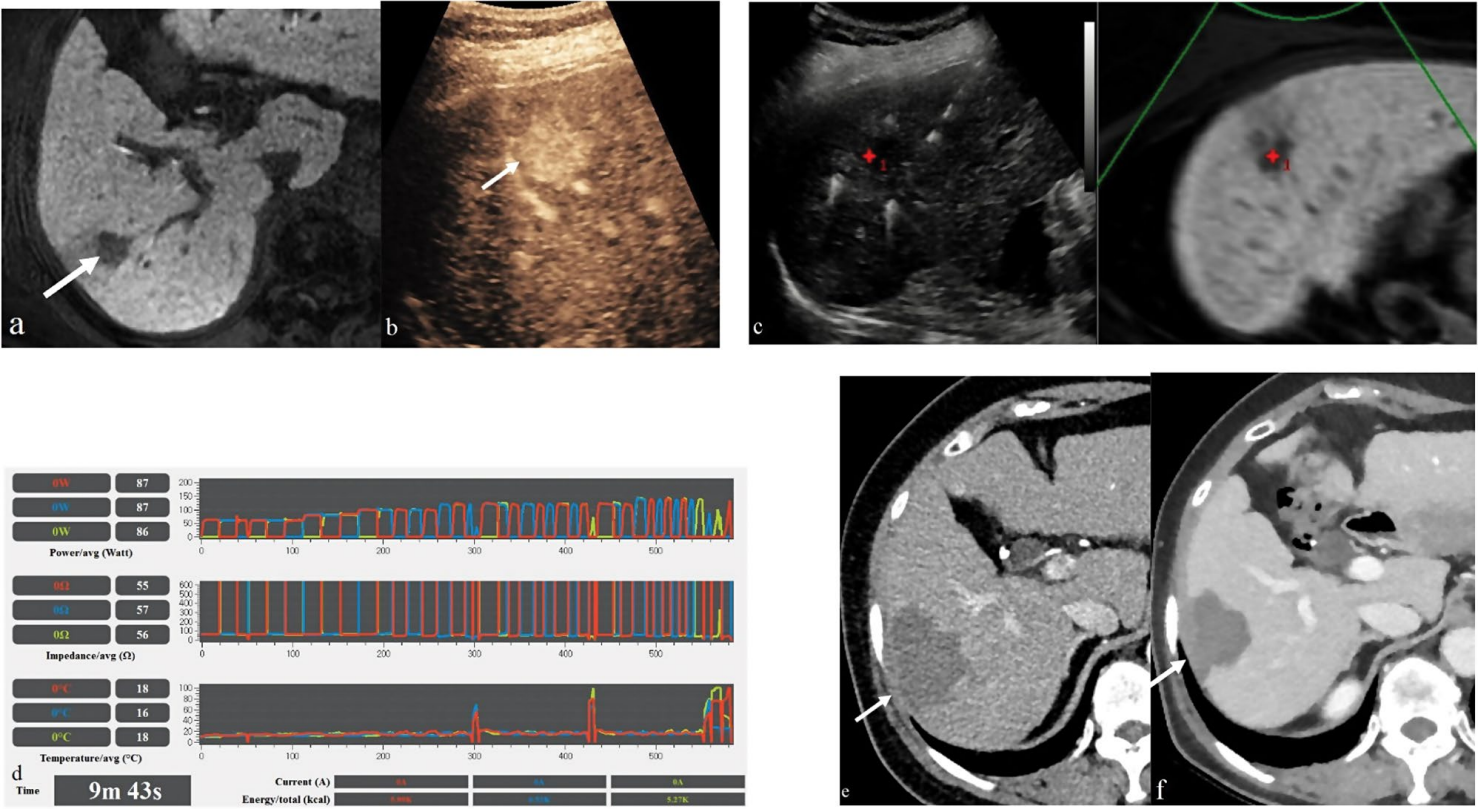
“No-tumor-touch” radiofrequency ablation in a 74-year-old woman with a 1.6-cm hepatocellular carcinoma (HCC) and hepatitis B-related cirrhosis. (a) Hepatobiliary phase image of gadoxetic acid-enhanced MRI shows a 1.6-cm low signal intensity HCC (arrow) in segment VI of the liver. (b) Contrast-enhanced ultrasound (Sonazoid) images well visualize the tumor (arrow). (c) Real-time ultrasound-computed tomography (CT)/magnetic resonance imaging (MRI) fusion imaging shows the low echoic target tumor. (d) In the panel, the active electrode was switched when the ablation time reached approximately 20–30 s. The electric current was initially set at 60 W and then systematically increased in 10 W increments every minute. The average impedance of each electrode was measured at 55–57 Ω, and this was correlated with the active electrode switch occurring when the impedance increased by 50 Ω above the baseline. The total ablation time was 9 min and 43 s, with the mean total energy of each electrode measured at 5.93 Kcal. (e) Portal venous phase coronal image of immediate CT scan shows complete ablation of the target tumor and tumor-bearing portal vein branches with a sufficient safety margin (> 5 mm). (f) No local tumor progression was observed at 39-month follow-up CT
